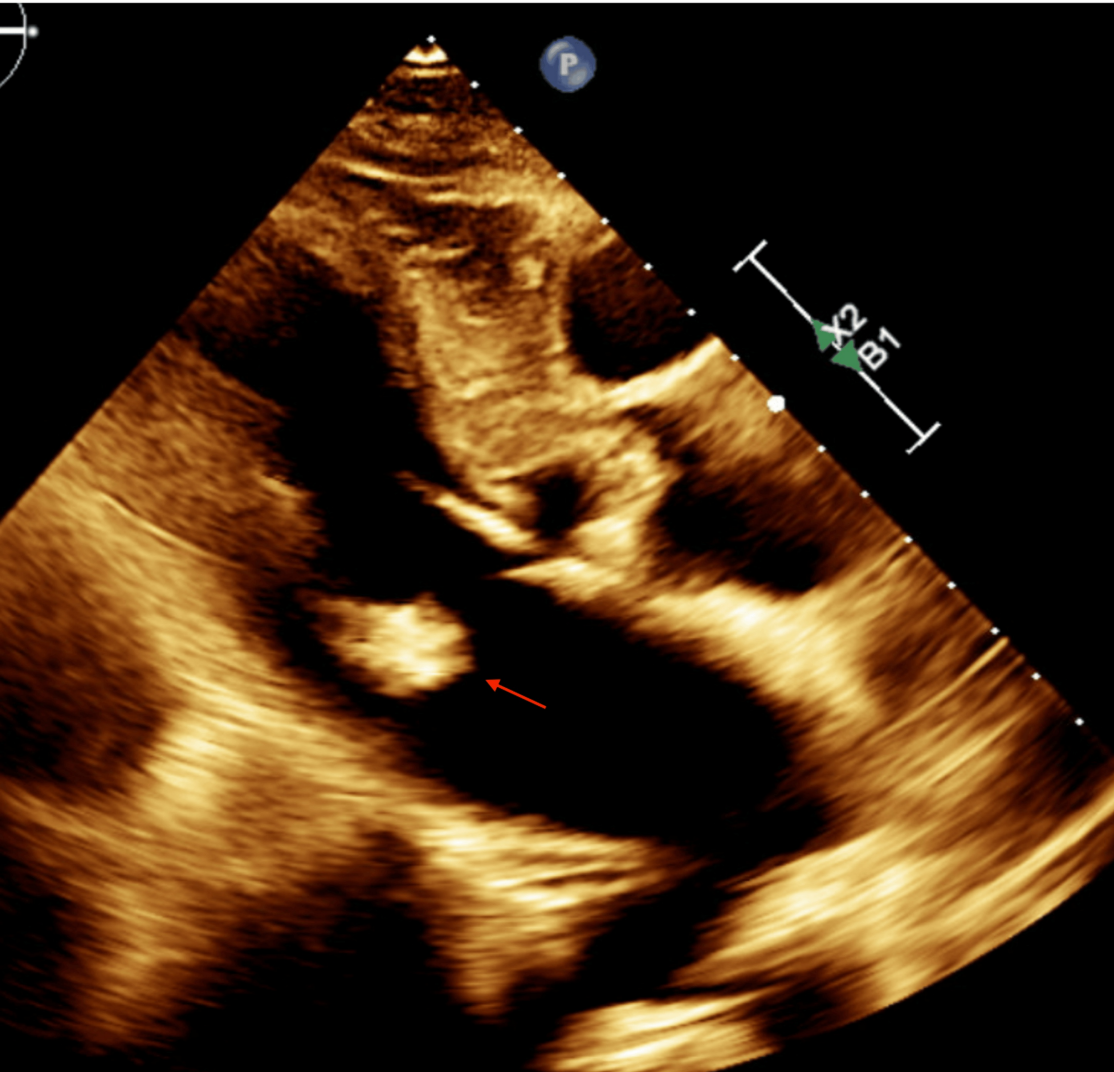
Two‐dimensional echocardiogram showing a calcified mass (red arrow) attached to the mitral annulus
